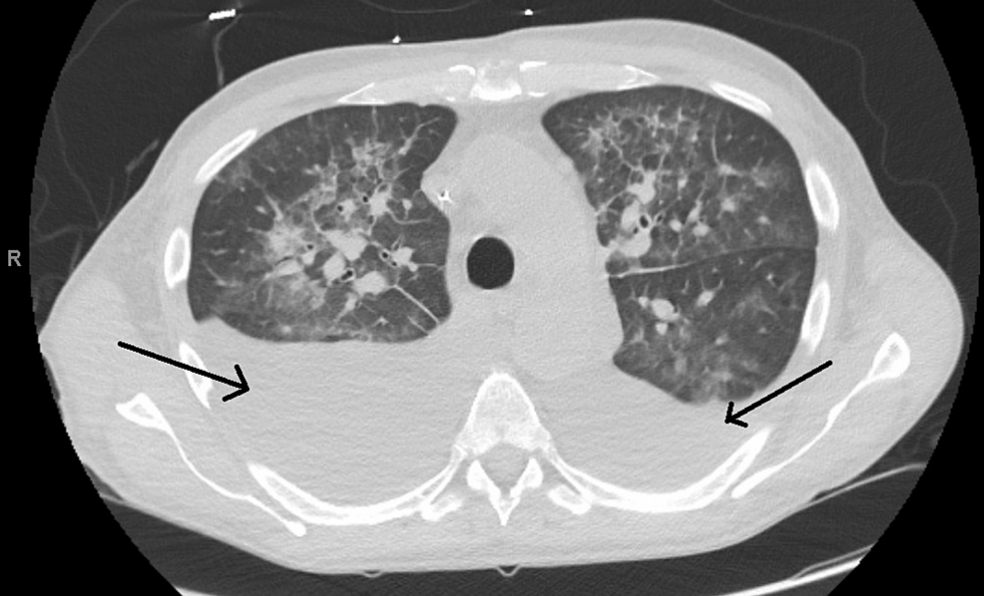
CT scan of the chest showing bilateral pleural effusions. CT: computed tomography
